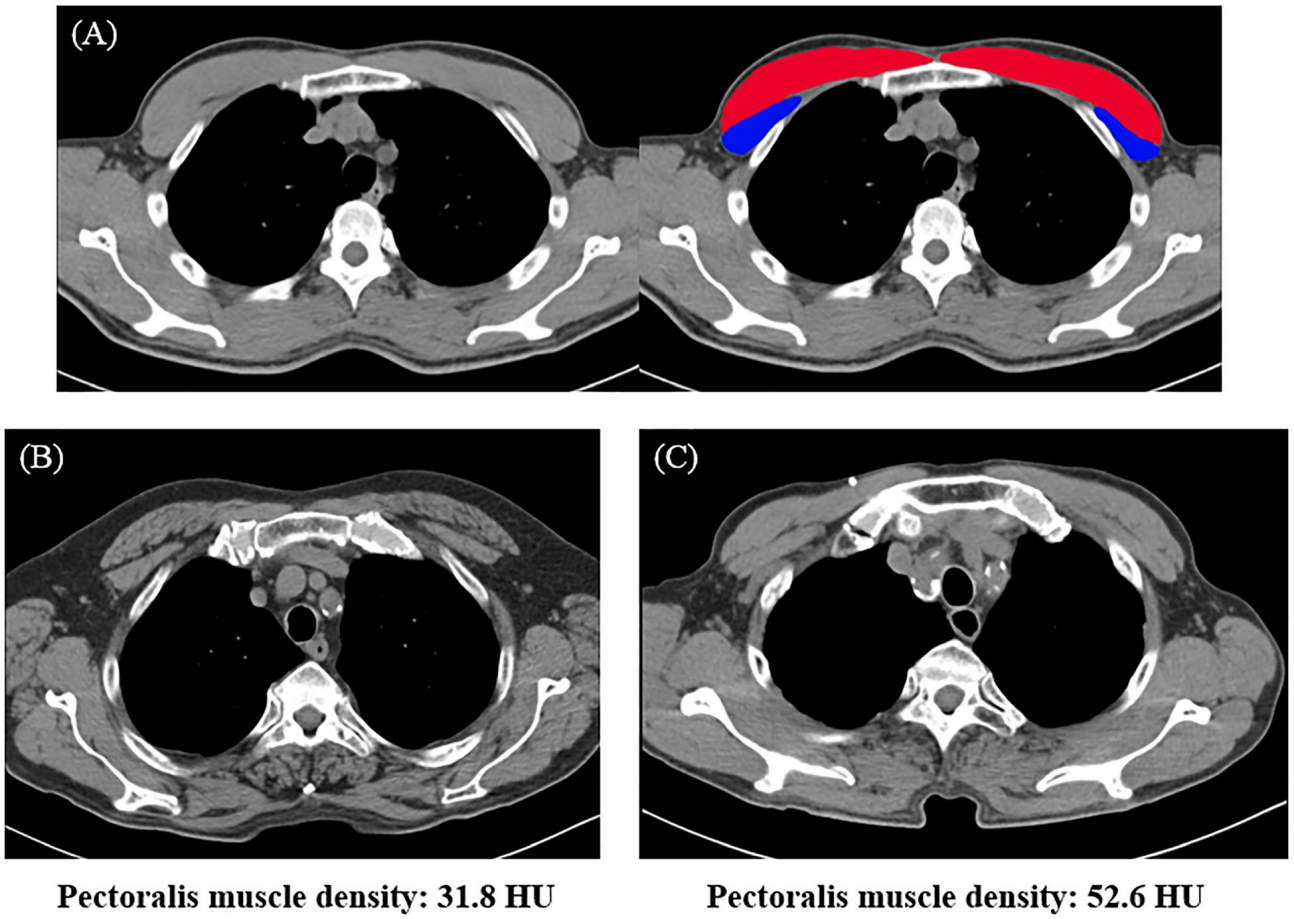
Computed tomography (CT) scans were used to assess the pectoralis muscle area and density. a, the CT axial slice above the aortic arch level shows the pectoralis muscle segmentation (red, pectoralis major muscle; blue, pectoralis minor muscle). b and c, differences between pectoralis muscle densities are depicted in CT images
